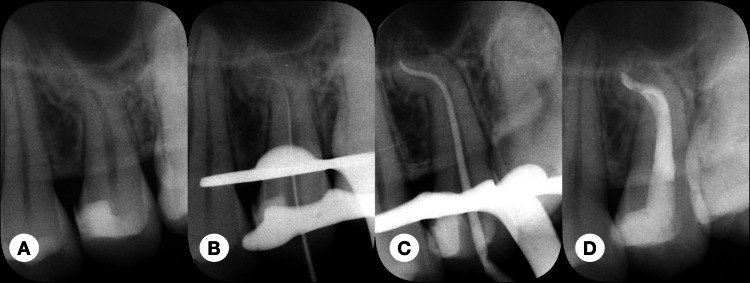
Pre-operative (A), working length confirmation (B), cone-fit (C), and post-obturation (D) radiographs of the maxillary left second premolar
